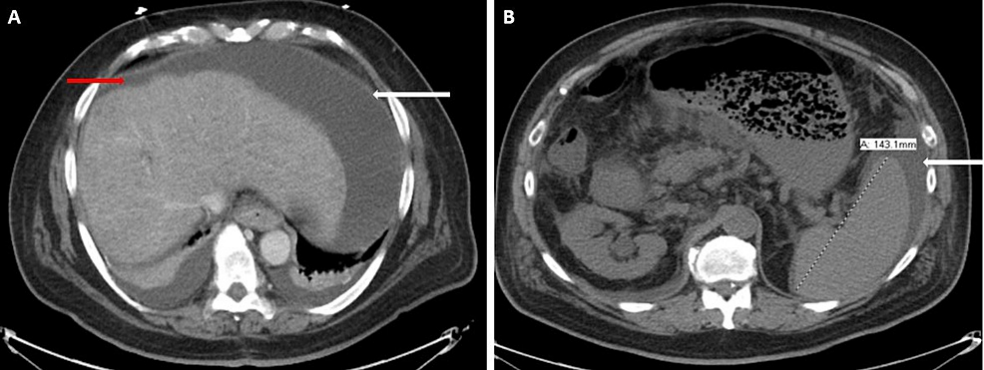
CT abdomen (selected upper abdominal axial sections) Figure 1A demonstrates irregular margins (red arrow) of the liver with nodular appearance and mild-to-moderate amount of free fluid (white arrow). Figure 1B depicts mild splenomegaly (splenic size 14.3 cm) with mild peri-splenic free fluid (white arrow).
